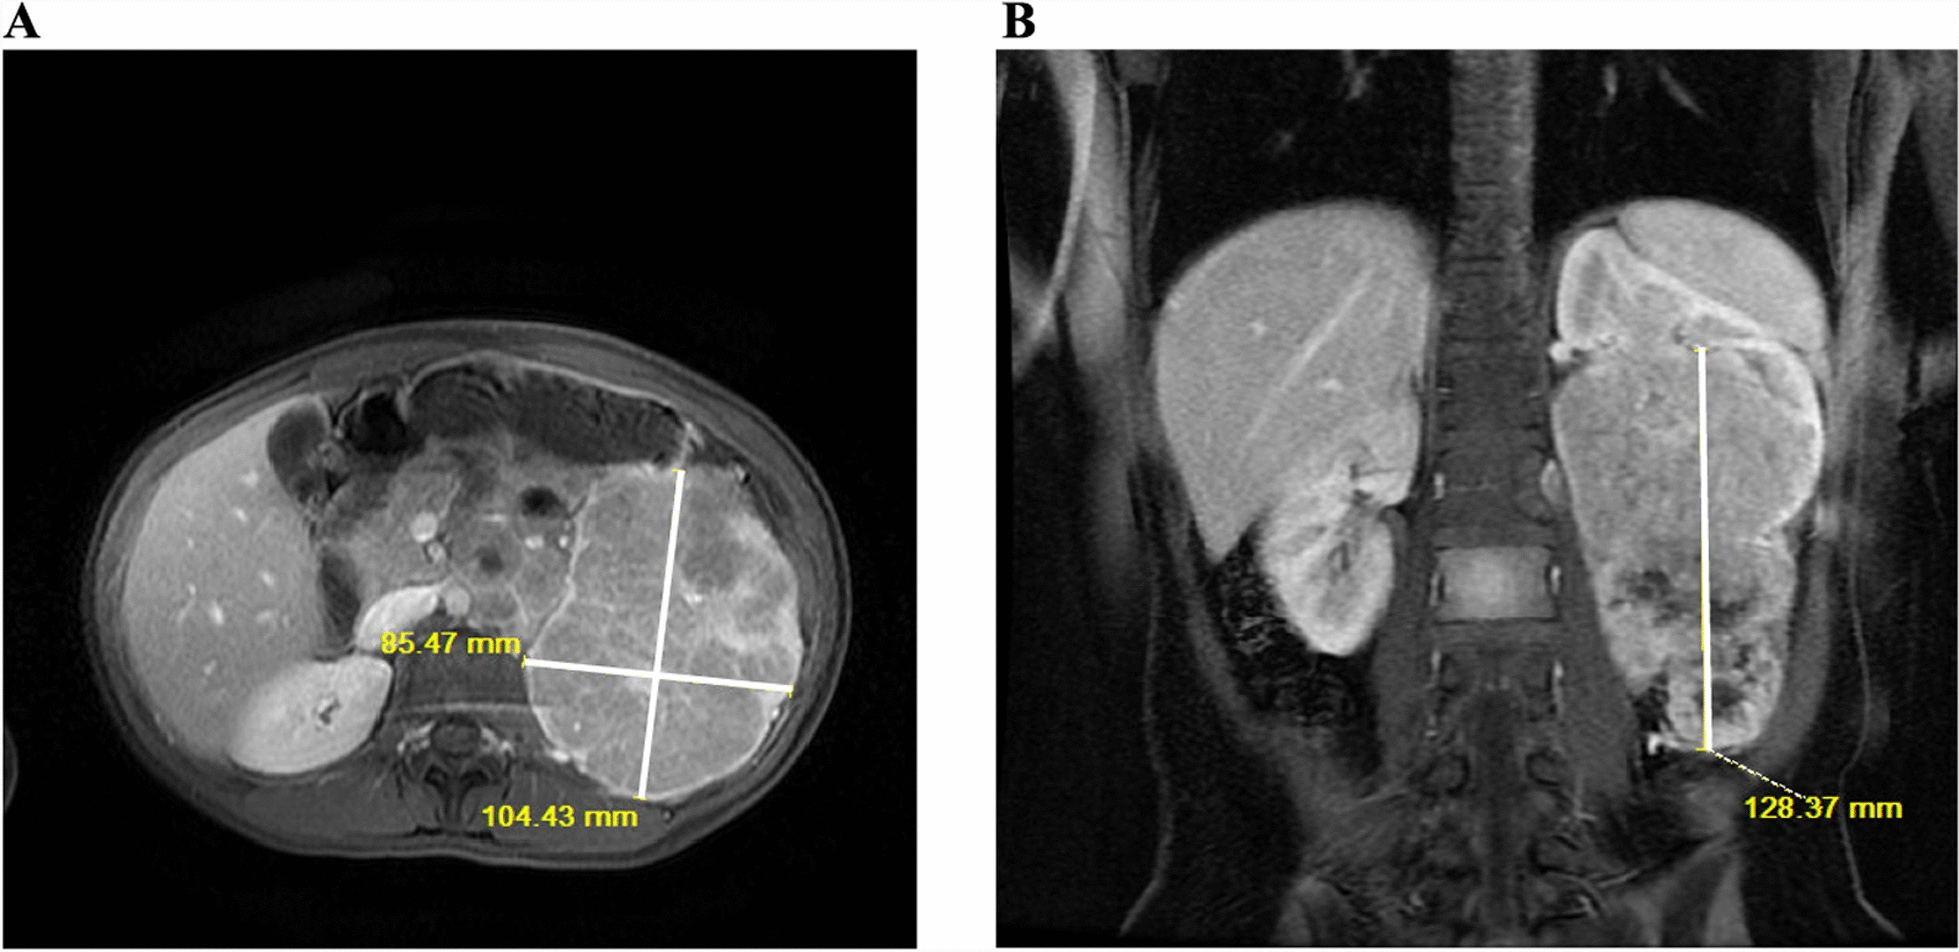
Magnetic resonance imaging of the abdomen and pelvis shows a large left-sided renal mass. (A) Axial and (B) coronal sequences are displayed
